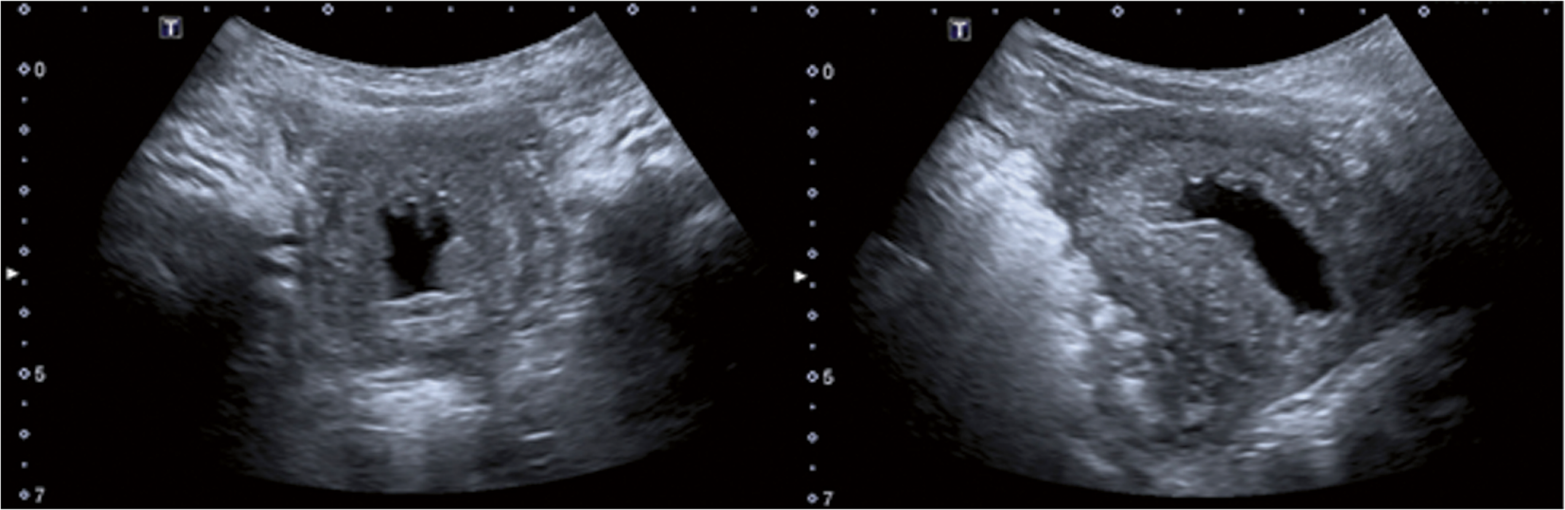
Sonography of the urinary bladder axial (left) and sagittal (right) displaying urinary bladder wall thickening (up to 2 cm) with heterogeneous echogenicity
